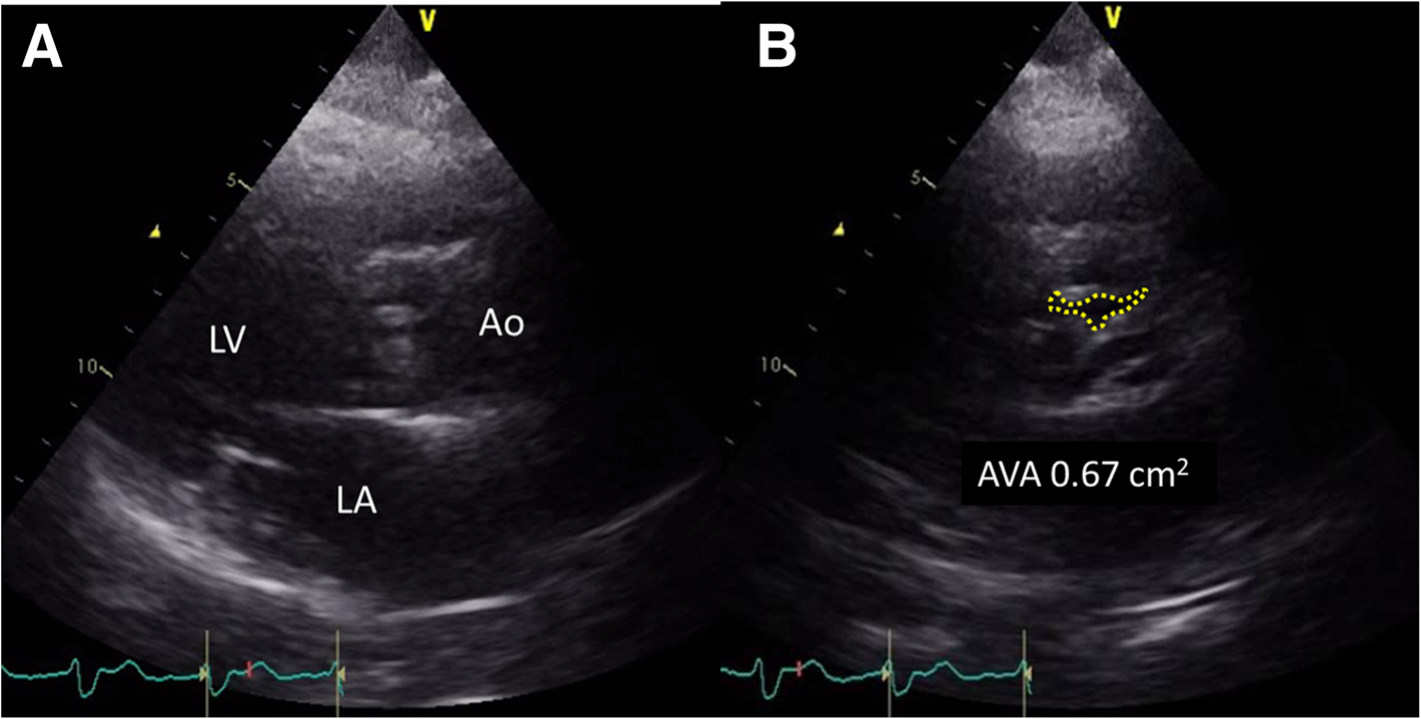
Pre-procedure transthoracic echocardiogram. a. Parasternal long axis view of severe aortic stenosis. b. Parasternal short axis view of 0.67-cm^2^ aortic valve, consistent with severe stenosis
